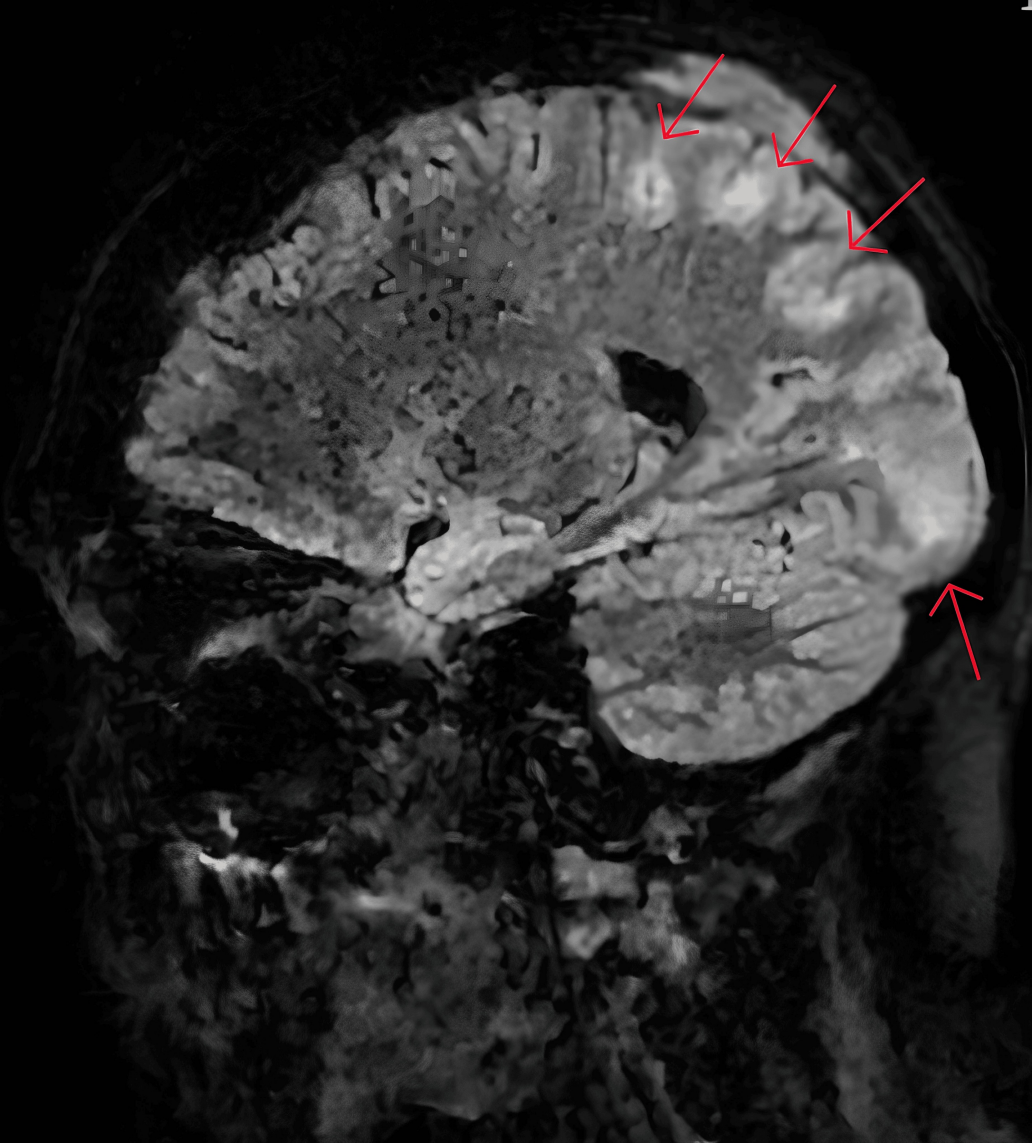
Sagittal FLAIR MRI slice Sub-cortical areas of high-signal intensity FLAIR: fluid-attenuated inversion recovery
